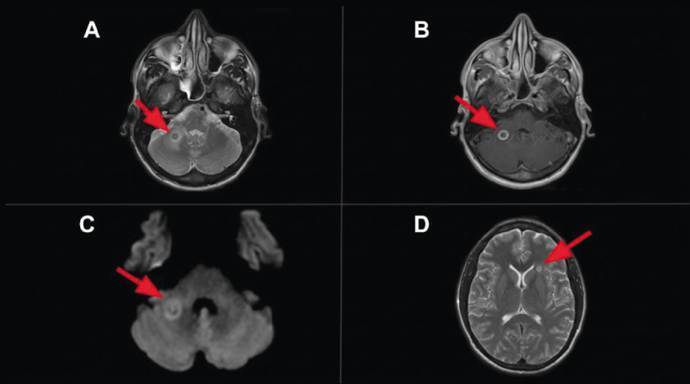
A: Axial T2-weighted magnetic resonance imaging (MRI) of the brain showing a well-defined intra-axial expansive lesion centered in the right cerebellar hemisphere (arrow), with a hyperintense central portion (visualized as a target-like area), and a hypointense peripheral zone. Perilesional vasogenic edema is also present. B: Axial T1-weighted MRI of the brain (imaged with a paramagnetic agent) showing peripheral enhancement of the lesion (arrow). C: Diffusion-weighted imaging showing an internal linear component and a peripheral area with restricted water diffusion (arrow). There was no diffusion restriction in the liquefied/necrotic region. D: Axial T2-weighted MRI of the brain showing a nodular lesion with a hyperintense periphery and hypointense center (arrow) in the left frontal periventricular white matter, but there was no perilesional edema.
